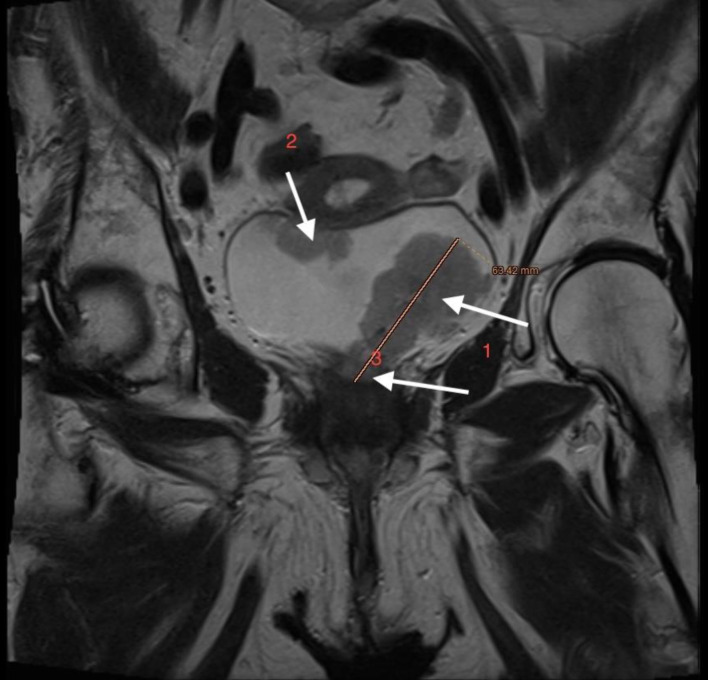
Lower abdominal magnetic resonance imaging (MRI) demonstrated an infiltrative tumor involving the urinary bladder and distal ureter (arrows 1 and 2), with extension into the uterus (arrow 3).
